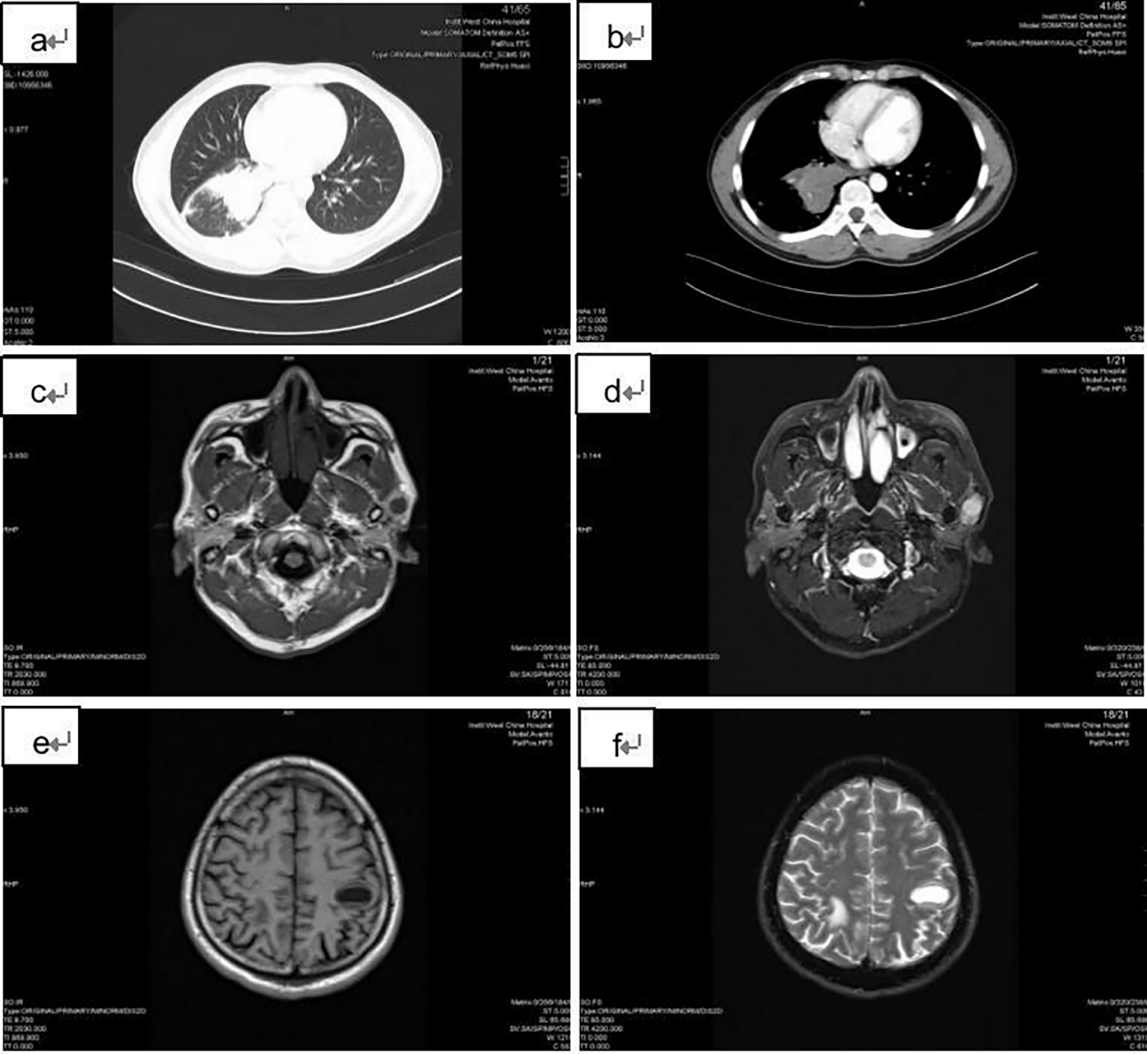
Imaging findings of the patient. (A) and (B): the chest CT showed a 6.4 cm x 5.2 cm mass at the right hilar; (C) and (D): T1 and T2 weighted head MRI images showed the preauricular mass located at the parotid gland; (E) and (F): T1 and T2 weighted head MRI images revealed the brain metastases.
